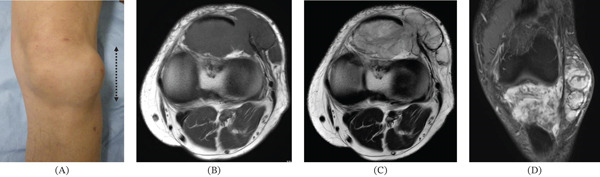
Findings at initial presentation and magnetic resonance imaging findings. (A) Findings on primary encounter. An elastic and firm tumor (black arrow: 10 cm) was evident in the left knee joint. (B–D) Magnetic resonance imaging findings. (B) Axial T1‐weighted spin echo. (C) Axial T2‐weighted spin echo. (D) T1 gadolinium‐enhanced image.
